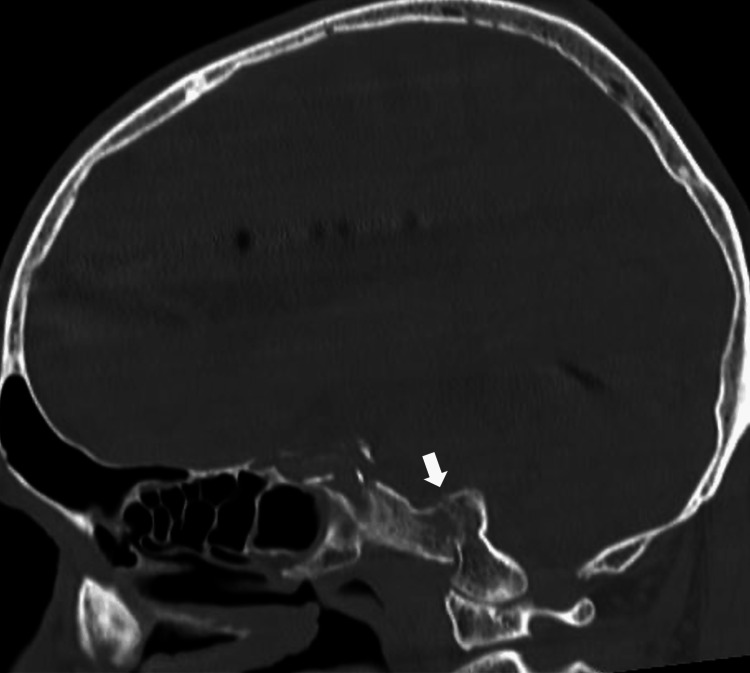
Sagittal-view bone-window computed tomography on hospital day 43. White arrow indicates porosis in the fractured clivus and consolidation of it, which suggests that the fracture is in the recovery process and becoming stable.
